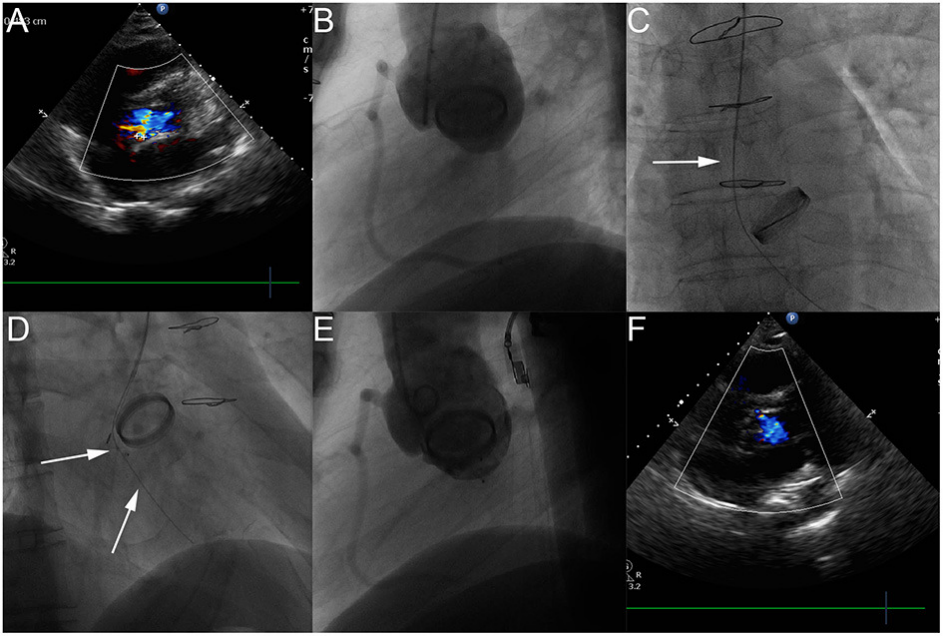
The process of transcatheter closure of aortic PVL. Transthoracic echocardiography (TTE) shows moderate aortic paravalvular regurgitation (A). The LAO 50° projection shows that moderate aortic paravalvular regurgitation (B). The AP projection shows that a hydrophilic guidewire was introduced retrogradely from ascending aorta (white arrow), crossing the aortic PVL, and finally going into the left ventricle (C). The RAO 30° shows that the first device was released through the delivery sheath over the first guidewire with the second hydrophilic guidewire leaving outside of the delivery sheath in the left ventricle (D). The LAO 50° projection shows that aortic paravalvular regurgitation disappeared (E). TTE shows that aortic paravalvular regurgitation disappeared after the procedure (F).
